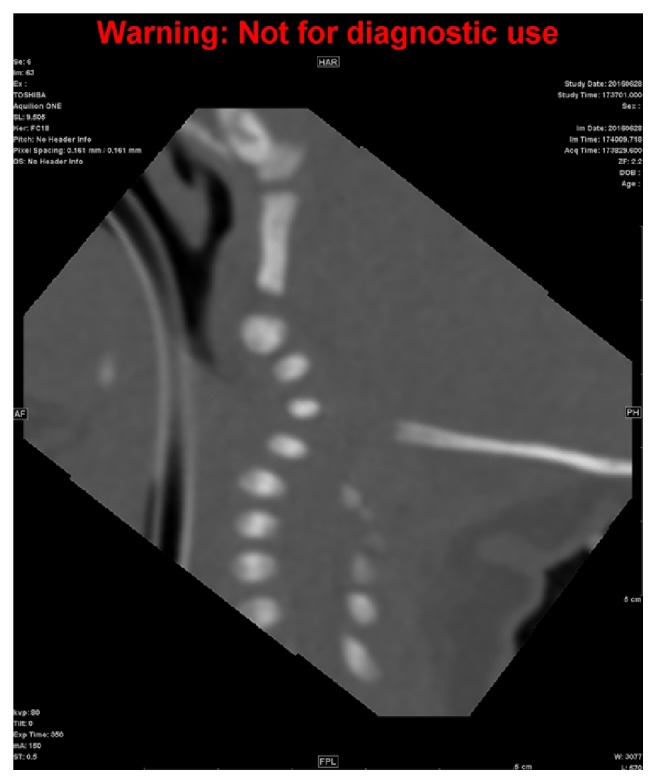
CT of cervical spine: occipitalization/assimilation of the atlas with severe kyphoscoliosis of the upper cervical spine, resulting in severe spinal canal stenosis with spinal cord compression. Incomplete/absent ossification centers of the upper cervical spine.
